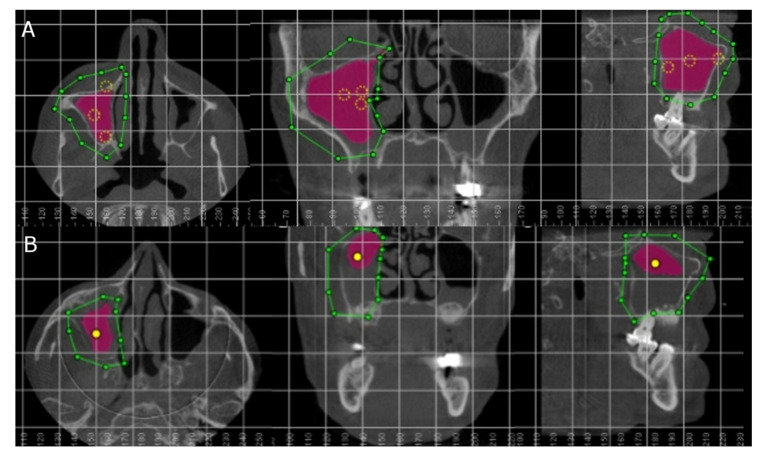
(A) Axial, coronal, and sagittal plane of the preoperative and (B) postoperative CBCT scans. Green line describes the selected area, yellow points define the air density, and purple area indicate the volume airway of the right maxillary sinus.
